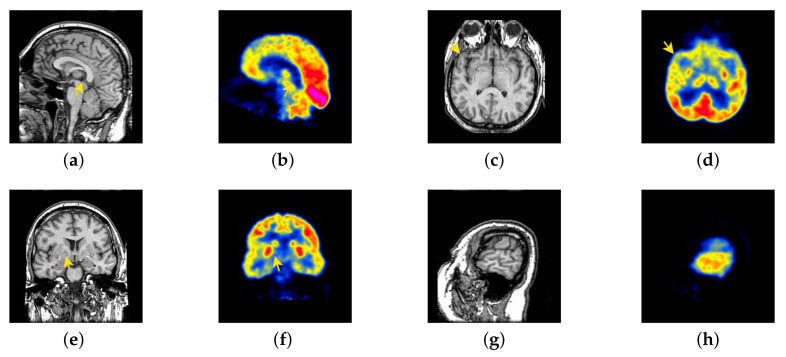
Four pairs of MRI-Fludeoxyglucose (FDG) source images: (a,c,e,g) are MRI images; (b,d,f,h) are FDG images.
